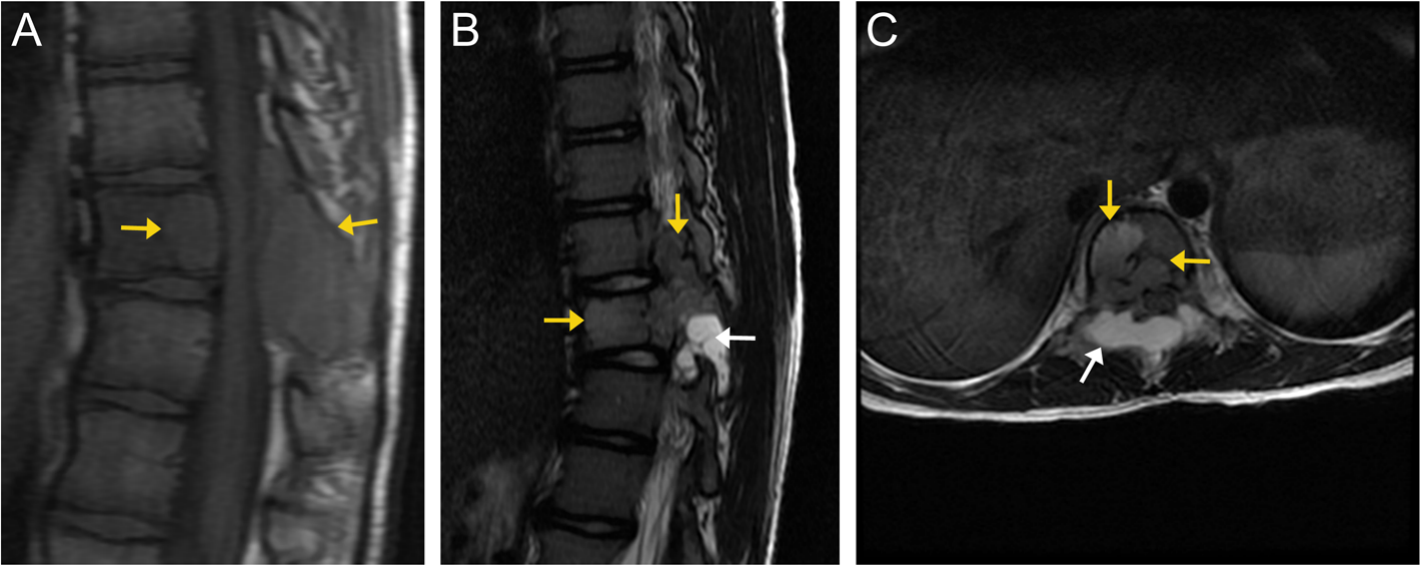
Osteoblastoma. Sagittal T1 weighted MR image (a) demonstrates a low signal lytic lesion involving the T10 vertebral body and posterior elements (orange arrows). The lesion is intermediate to high signal on T2 weighted images (b, c). There is an associated T2 hyperintense component, consistent with an aneurysmal bone cyst (white arrow), which can be seen in 10-20% cases of osteoblastomas
